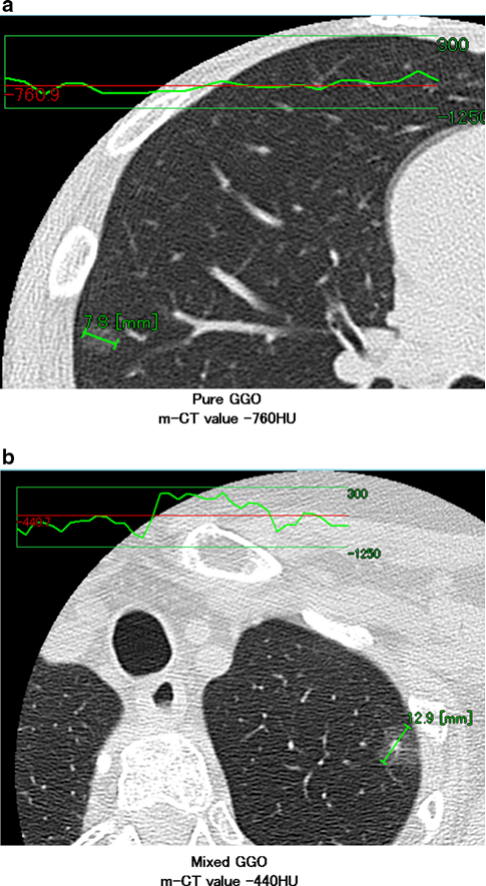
The CT image with a profile of the density across the tumor. The profile of pure GGO revealed an almost flat curve, and the m-CT value was calculated to be −760 HU (a). The profile of mixed GGO revealed that the CT value of the solid portion was higher than the GGO portion, and the m-CT value was calculated to be −440 HU (b)
